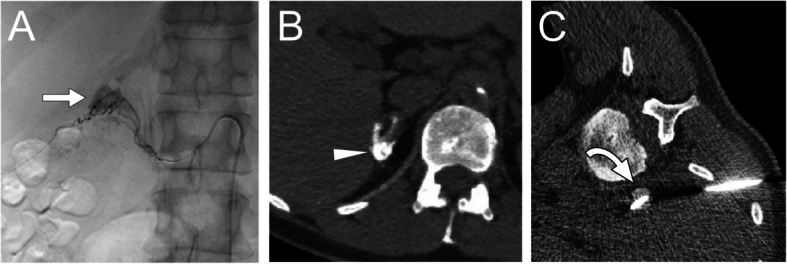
Lipiodol marking and RFA for right adrenal adenoma (Case 1). A Right adrenal arteriography from the microcatheter revealed contrast in the adrenal adenoma (arrow) and parenchyma. B Adrenal computed tomography (CT) image after lipiodol-marking. Lipiodol is deposited in almost of the adenoma (arrow) and part of the adrenal parenchyma. C CT fluoroscopy image during radiofrequency ablation. The high-density adenoma for lipiodol (arrow) is easy to visualize under the insertion of two thick needles. This image parameters were 120 kVp, 20 mAs, and three slices 2 mm thick
